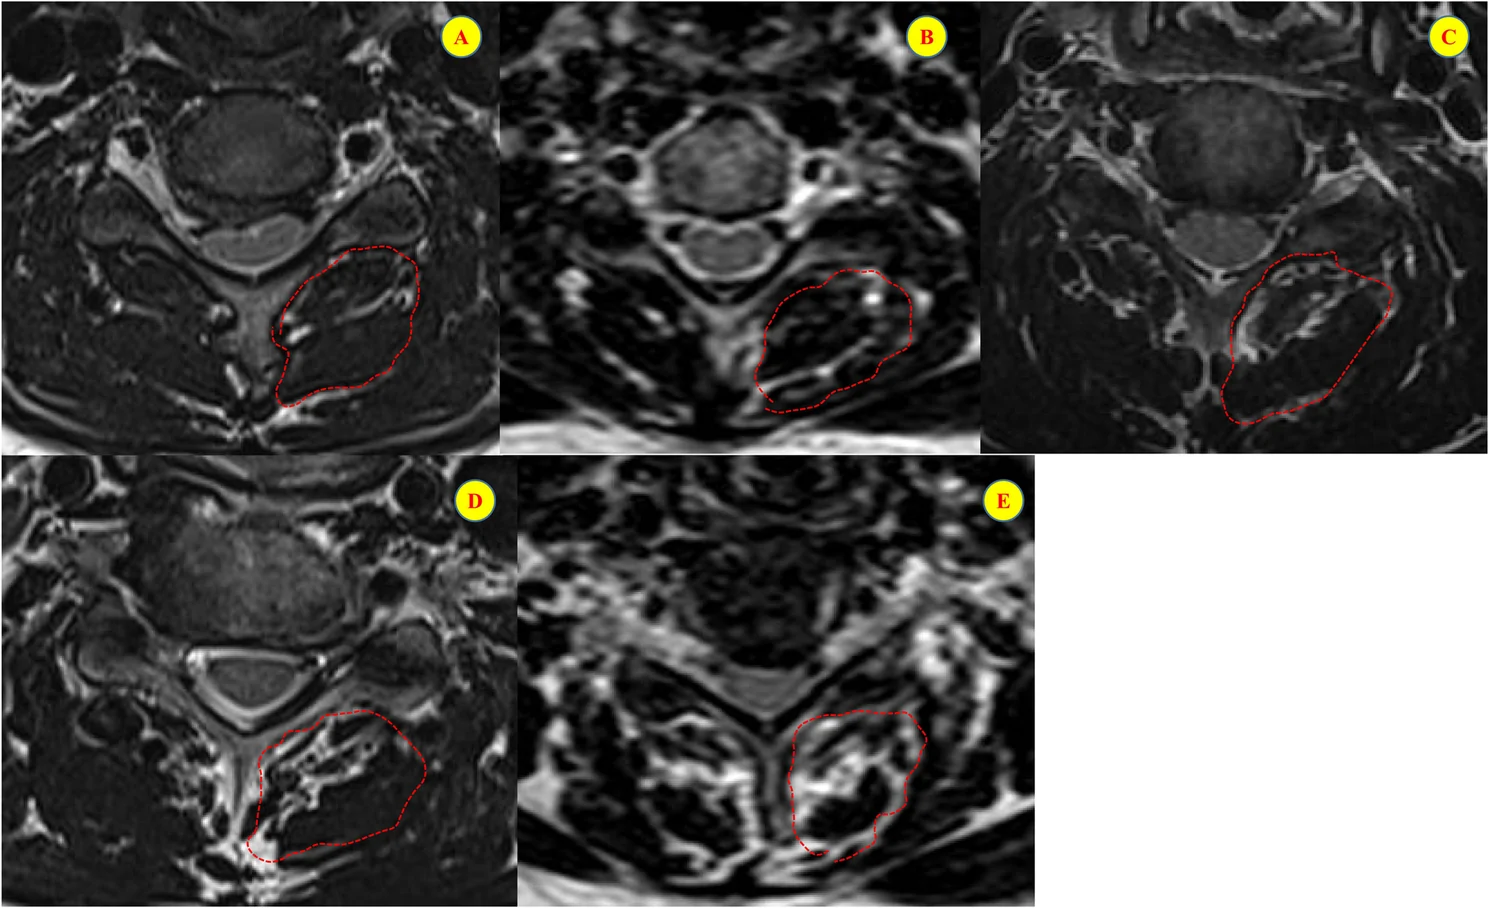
T2-weighted axial images obtained at the C5–6 intervertebral level, used to assess and grade paraspinal muscle fat infiltration. Panels (A), (B), (C), (D), and (E) correspond to grades 0, I, II, III, and IV fat infiltration, respectively
